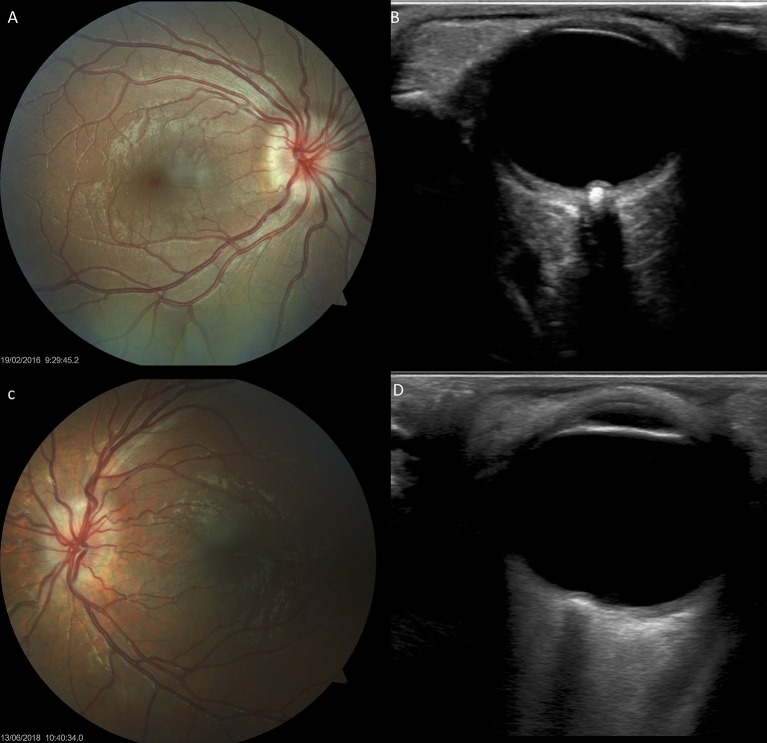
Optic disc drusen in children with Down syndrome, as evidenced by fundus imaging and ocular B-scan ultrasound. (A,C) Optic disc drusen in two children with Down syndrome; a spoke-like vessel pattern is evident in (A). The optic discs appear smaller, with no physiological cupping. (B,D) Ocular B-scan ultrasound in the same patients, showing ovoid echogenic lesions, with acoustic shadow, at the junction of the retina and the optic nerve.
